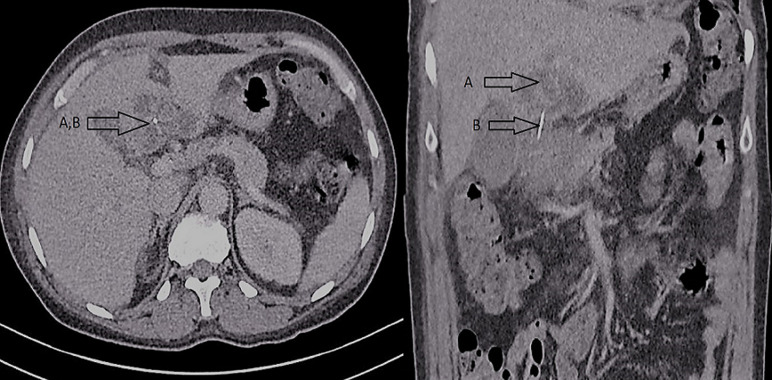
CT scan performed on admission, showing the septated segment III/IVb liver abscess (A) and the fishbone (B)
